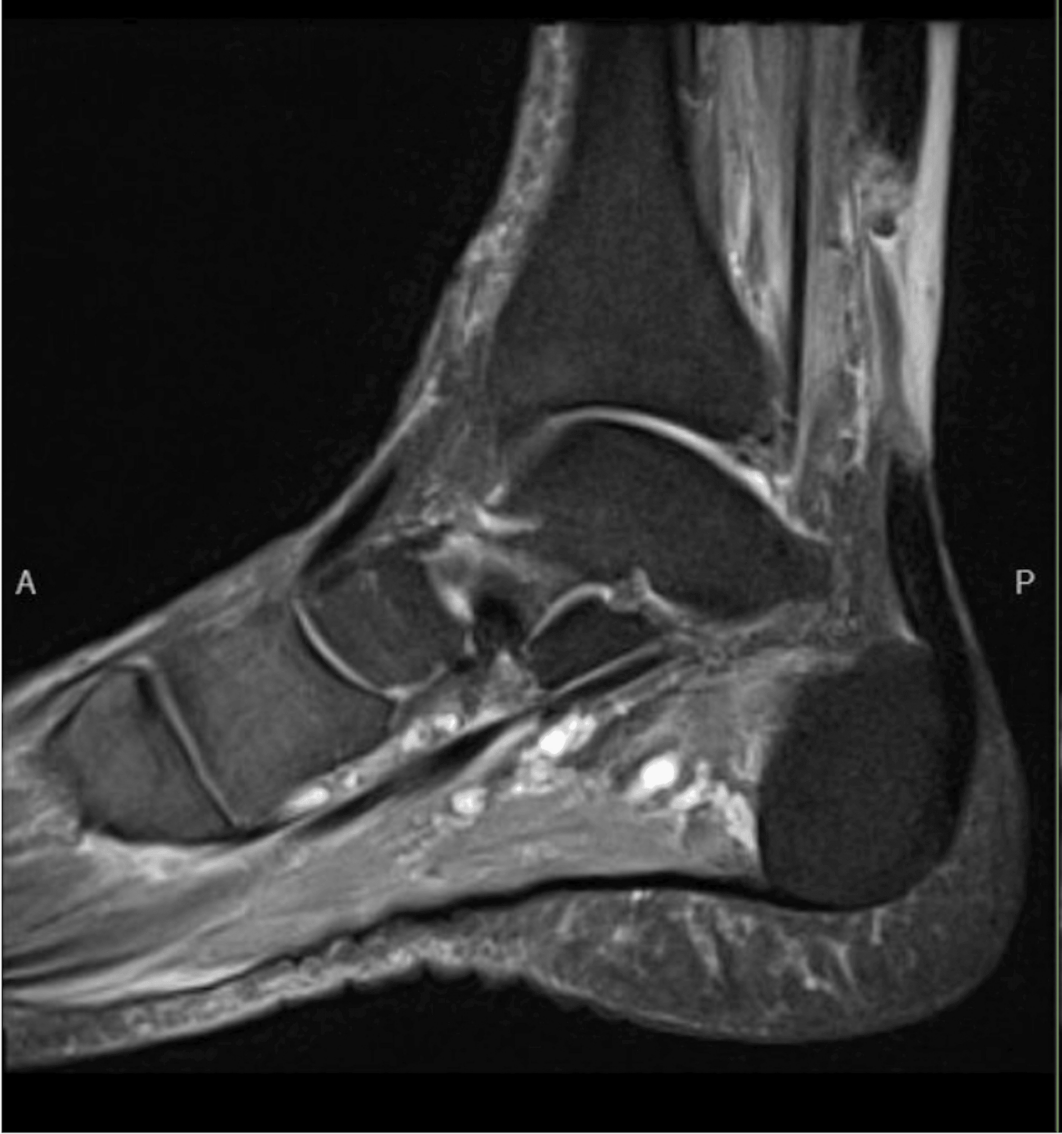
Achilles tendon (left) sagittal PD MRI image PD: proton density; MRI: magnetic resonance imaging
